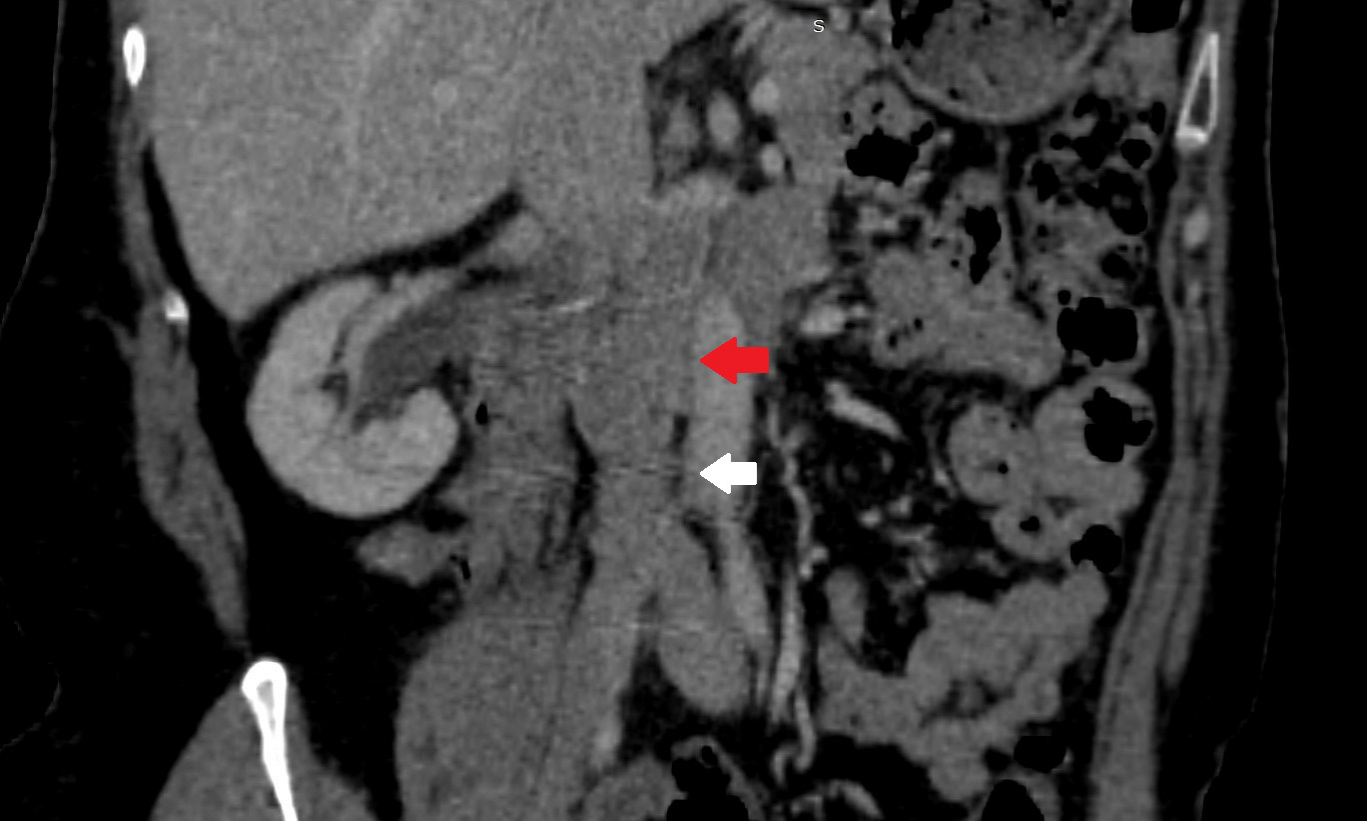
Coronal computed tomography angiography of the abdomen and pelvis in the fifth postoperative month. Red arrow indicates the Dacron graft; white arrow indicates the distal anastomosis with the inferior vena cava.
